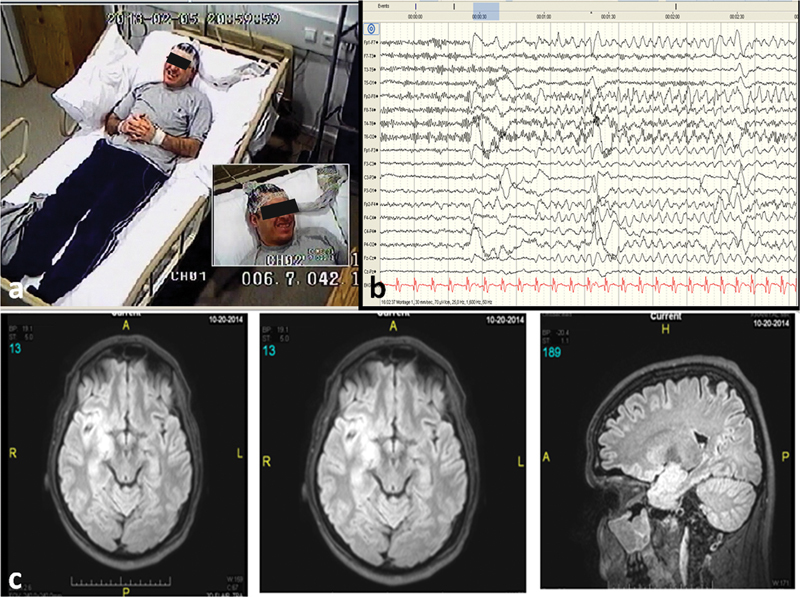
Patient 20: a) Smile, awareness and automatisms, b) ictal EEG: F8-T4 spike wave activity, c) cranial MRI, right temporal dysplasia.
